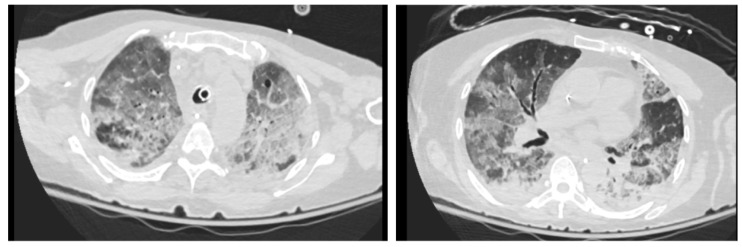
CT scan on the 10th day of admission. CT scan on the 10th day of admission, a “crazy paving” pattern markedly increased in severity. Increase in component of consolidative opacities, predominantly peribronchovascular and regions of ground-glass, air bronchogram seen in the lesion, no discrete cavitation.
